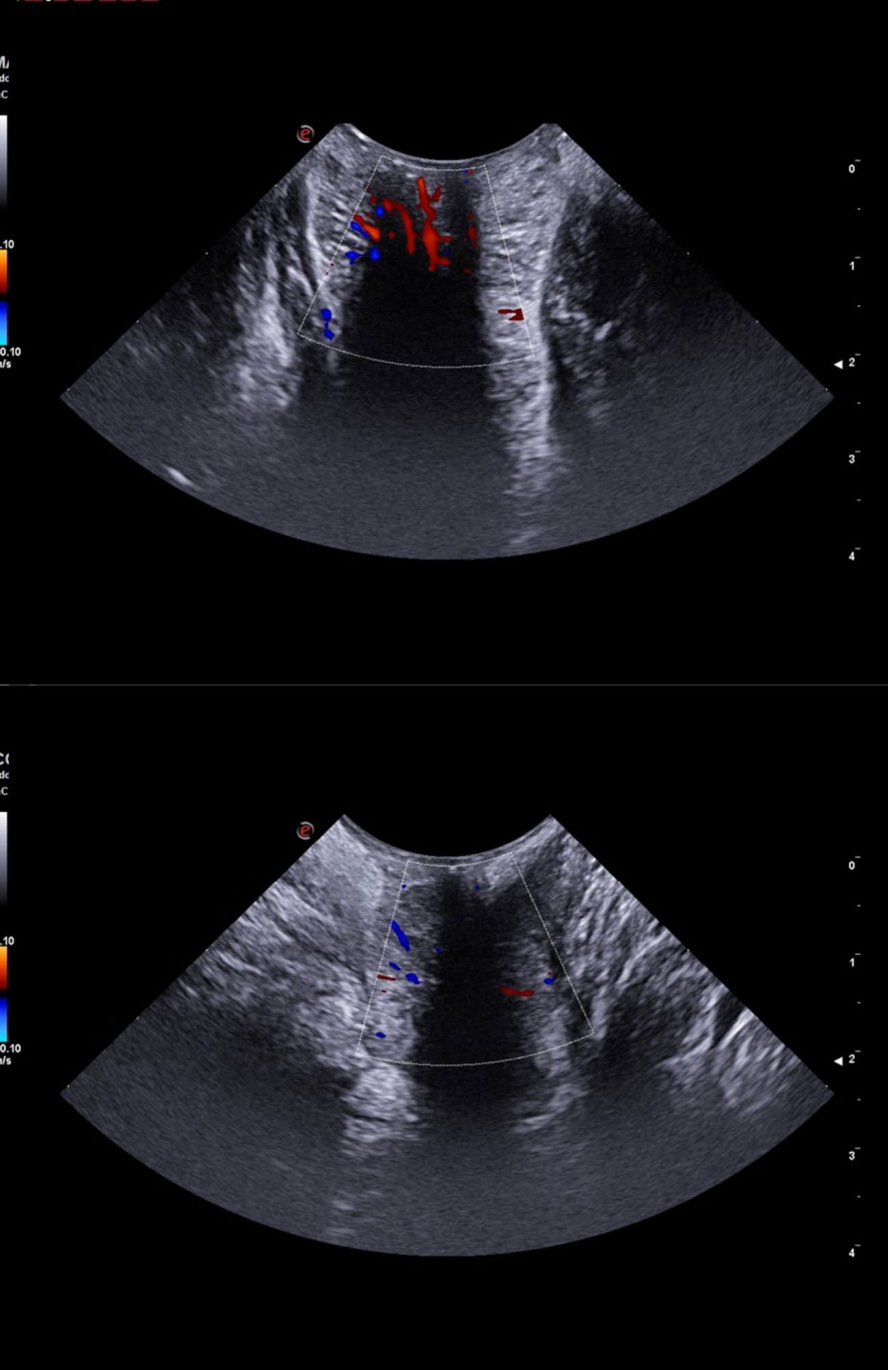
Patterns of Doppler vascularity: vascular (*top panel*) and scattered (*bottom panel*)
